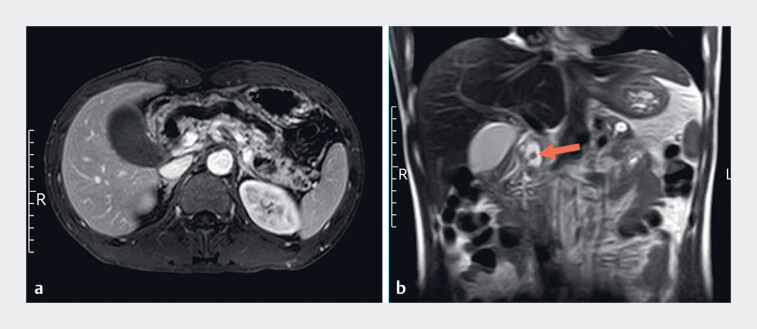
Magnetic resonance imaging (MRI) showed chronic pancreatitis with main pancreatic duct stones in a 33-year-old man with intermittent abdominal pain.
